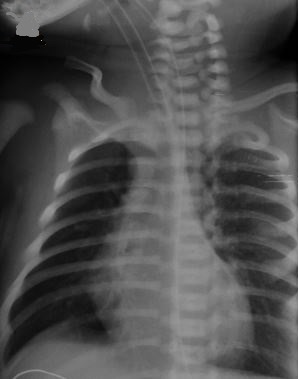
Egg on a string sign in chest X-ray
